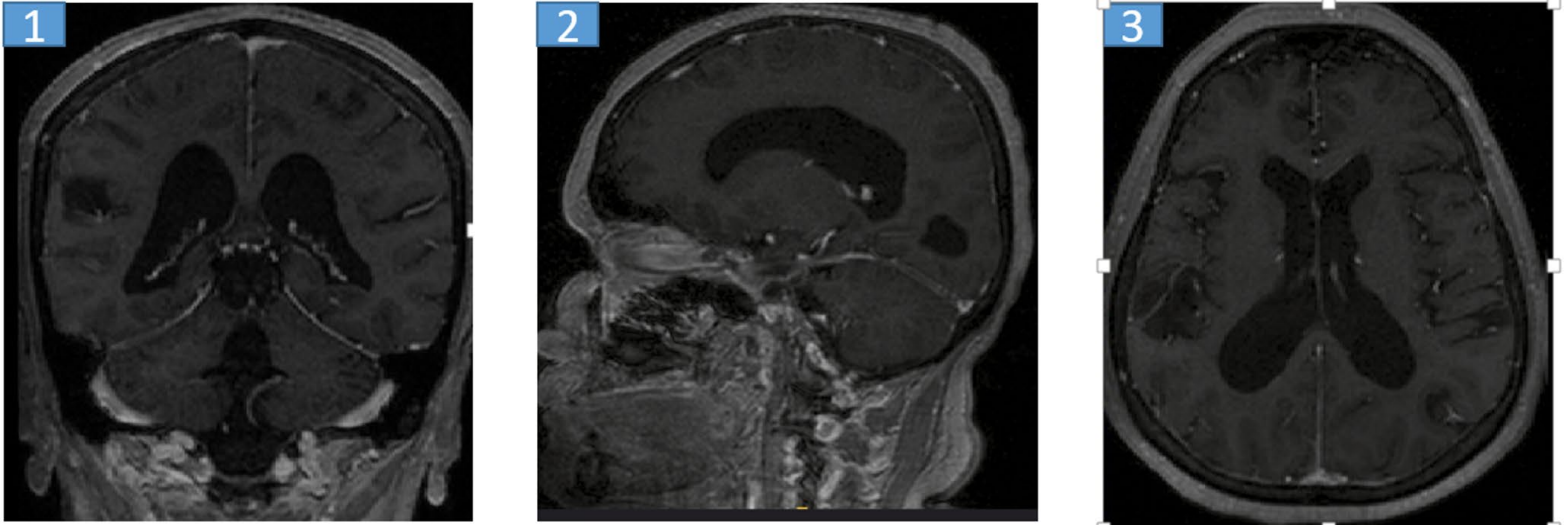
MRI scan of the brain in a 50-year-old female patient diagnosed with a tumor 1: Coronal MRI image of the brain with tumor. 2: Sagittal MRI image of the brain with tumor 3:Axial MRI image of the brain with tumor
